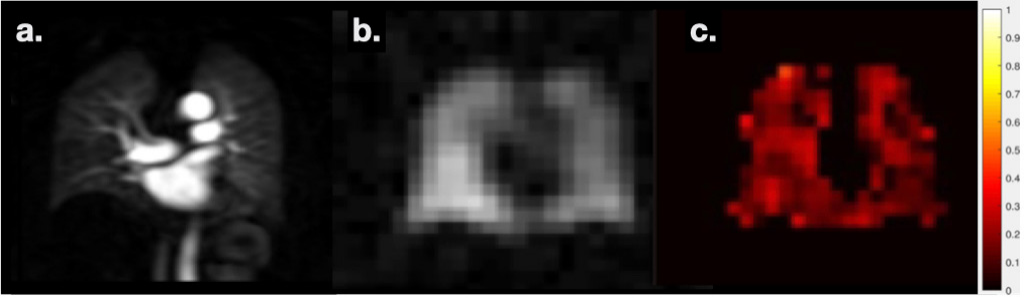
Patient J: Referred around ten months after having COVID-19. The patient had an acute infection post-COVID and persistent breathlessness; “long-COVID”. ^1^H structural and perfusion imaging revealed no apparent abnormalities (a). HP ^129^Xe ventilation imaging showed a homogeneous pattern of ventilation (b) with no substantial defects, however, significant gas transfer impairment was indicated by a mean RBC/TP (mean of map in c.) of 0.15 (c.f. healthy ~0.47) and elevated TP/Gas. Of topical note, preliminary reports of HP gas MRI in patients post-COVID-19 have been recently published^
